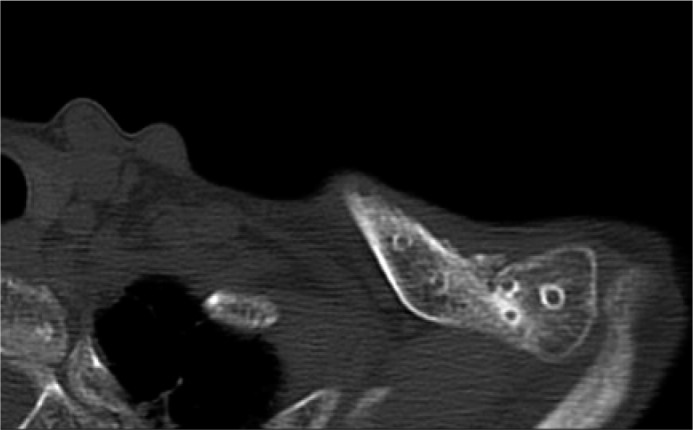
CT scan image after implant removal at 14 months after operation. Bony union was achieved.
